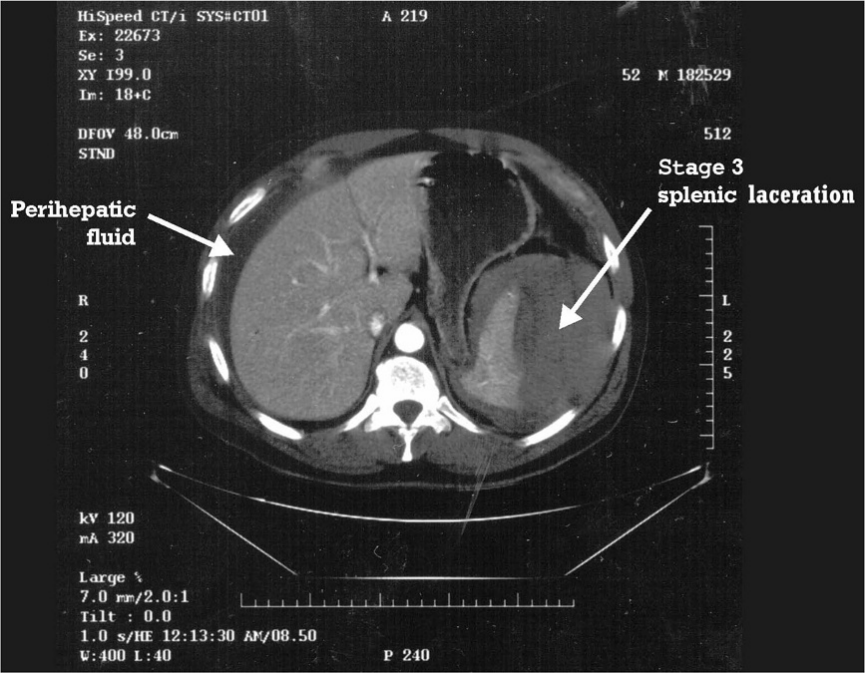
CT scan: stage 3 splenic injury with hemoperitoneum.
